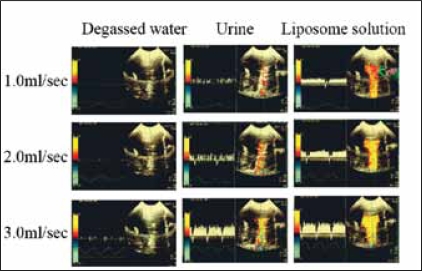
Comparison of color Doppler signals among four flow materials. With degassed water Doppler signals could not be detected on the display at any flow rate (0.3-4.0 ml/s). At 1.0 ml/s no Doppler signal was detected from distilled water or urine. Continuous flow signals were detected using the liposome solution at flow rate 0.3 ml/s or higher. At 2.0 ml/s flow rate, continuous flow signals were obtained from urine and liposome solution
